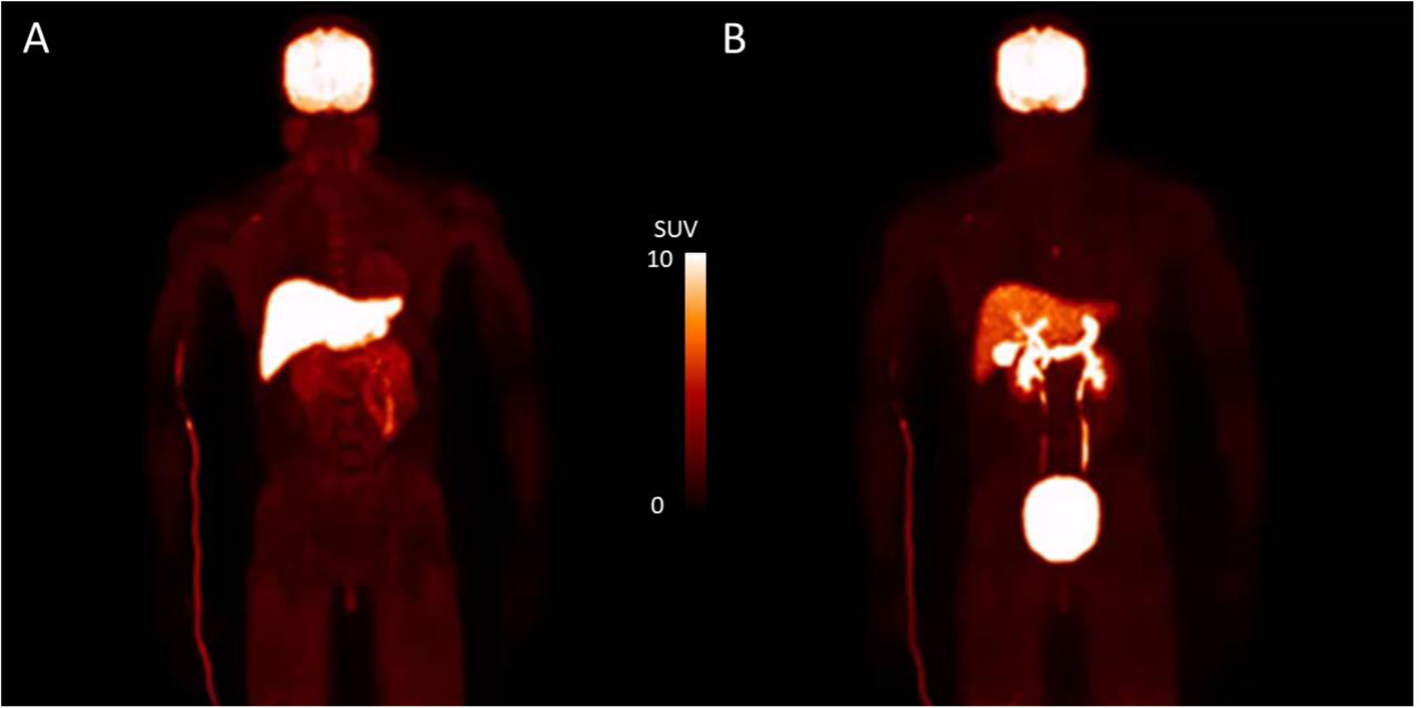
Representative maximum intensity projection images of ^11^C-UCB-J: a 2–10 min, b 30–60 min. SUV scale, 0–10
